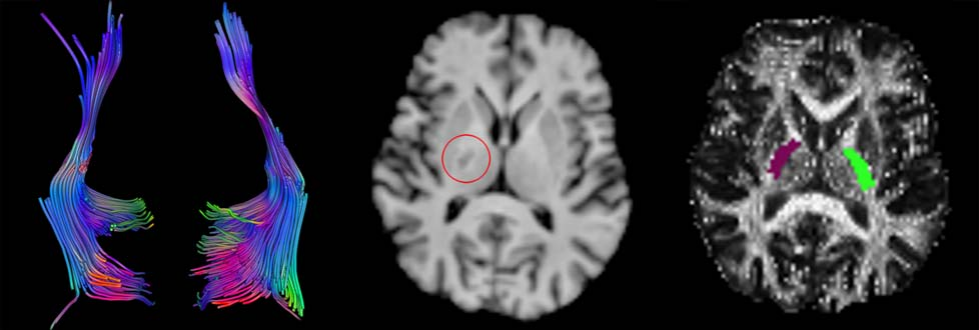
Diffusion tensor tractography in a stroke patient. The results of diffusion tensor tractography (left) in a patient who experienced subcortical stroke, causing relevant unilateral hand motor impairment. The lesion in the left posterior limb of the internal capsule is marked by a red circle on a T1-weighted anatomical slice image (middle), the de-normalized ROIs are shown as colored overlays on an FA slice image (right).
